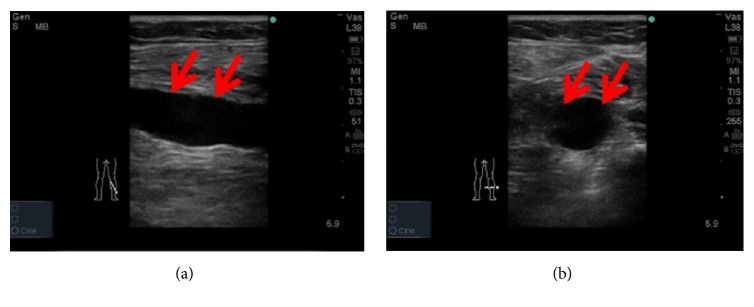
Ultrasound screening of dilated soleal vein (SV). (a) shows hypoechoic signals in the longitudinal sections of calf on B-mode screening (red arrows); (b) shows a round hypoechoic area was apparent on the transverse section (red arrows).
